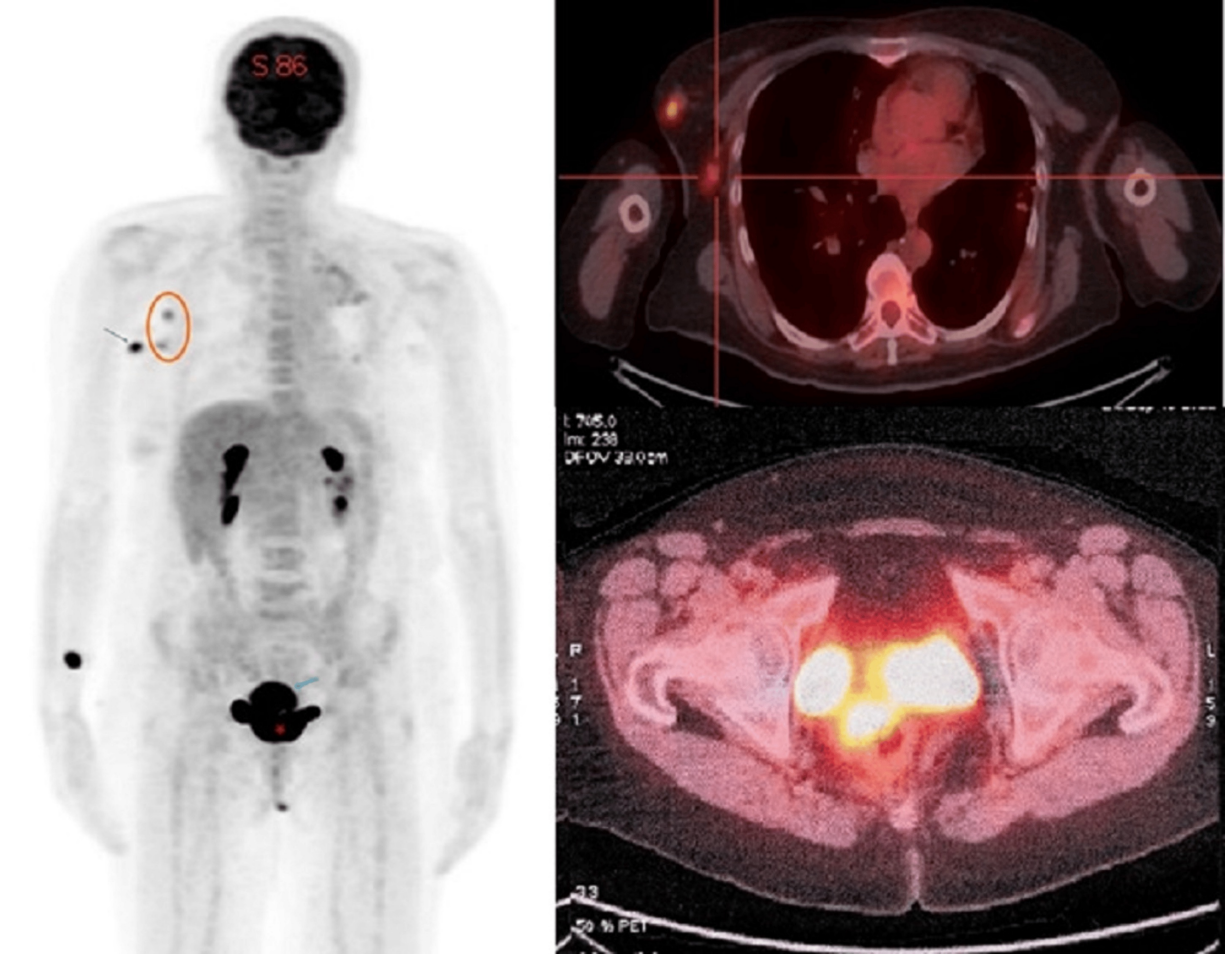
The 18F-FDG PET/CT shows simultaneous hyperfixation of the breast nodule with ipsilateral axillary lymphadenopathy and the cervical mass, without evidence of suspicious distant lesions.
